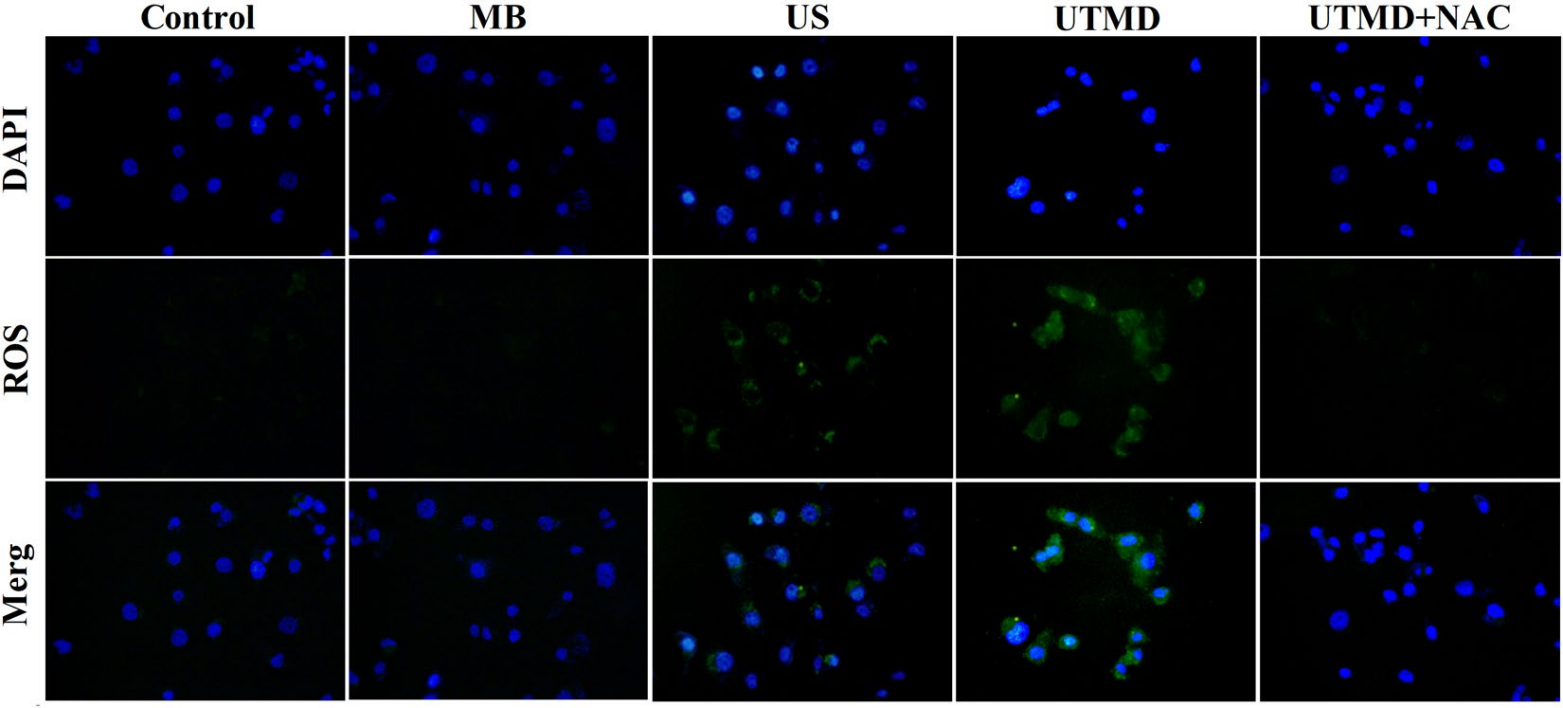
Representative fluorescent images of production of DCF due to ROS by the MDA231 cells after various conditions of stimulation. “Control” represents the group without treatment, “MB” represents the group with microbubble, “US” represents the group with ultrasound treatment, “UTMD” represents the group with UTMD treatment, and “UTMD + NAC” represents the group with NAC pre-incubation before the UTMD treatment.
